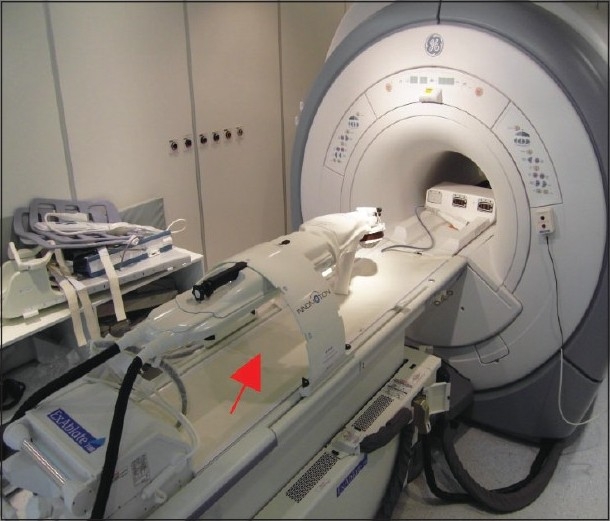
Robotic-assisted MRI-guided HIFU system (note robot-red arrow)
